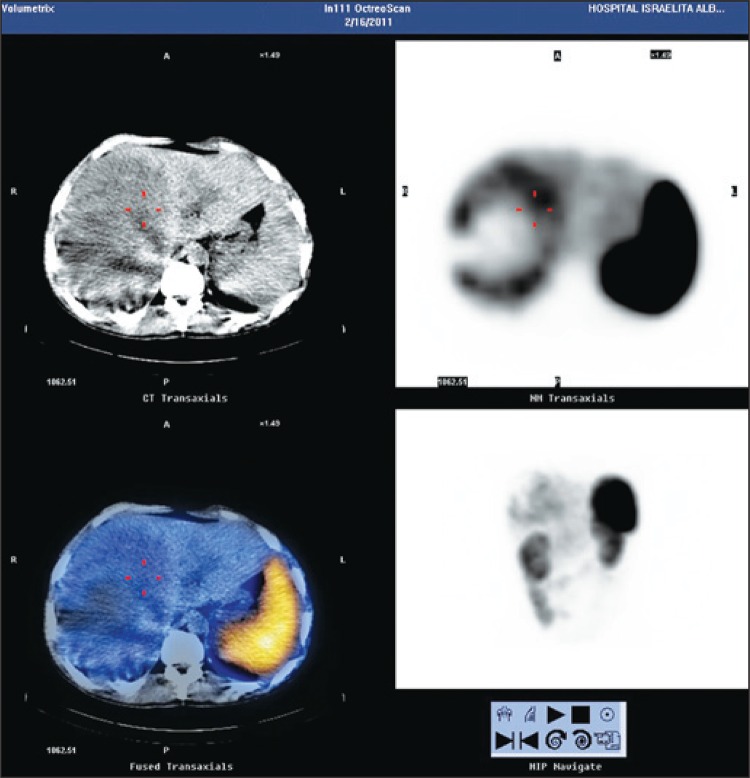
Octreotide scintigraphy shows radiomarker uptake in hepatic lesion
